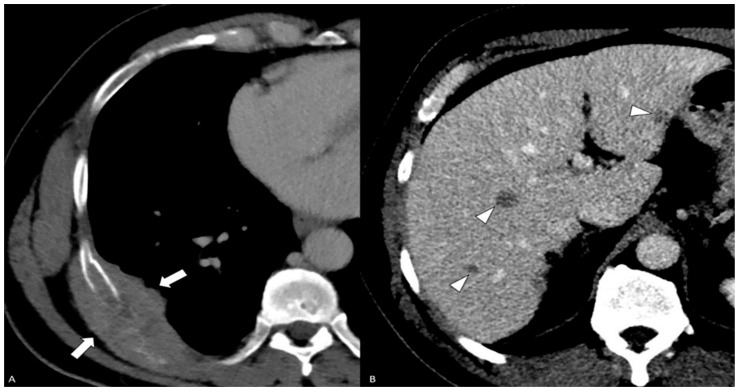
Contrast-enhanced CT—(A) axial post-contrast CT of the chest shows an expansile right 8th rib mass with a subpleural component (white arrows). (B) Axial post-contrast CT of the abdomen shows multiple small ill-defined liver lesions (white arrowheads).
